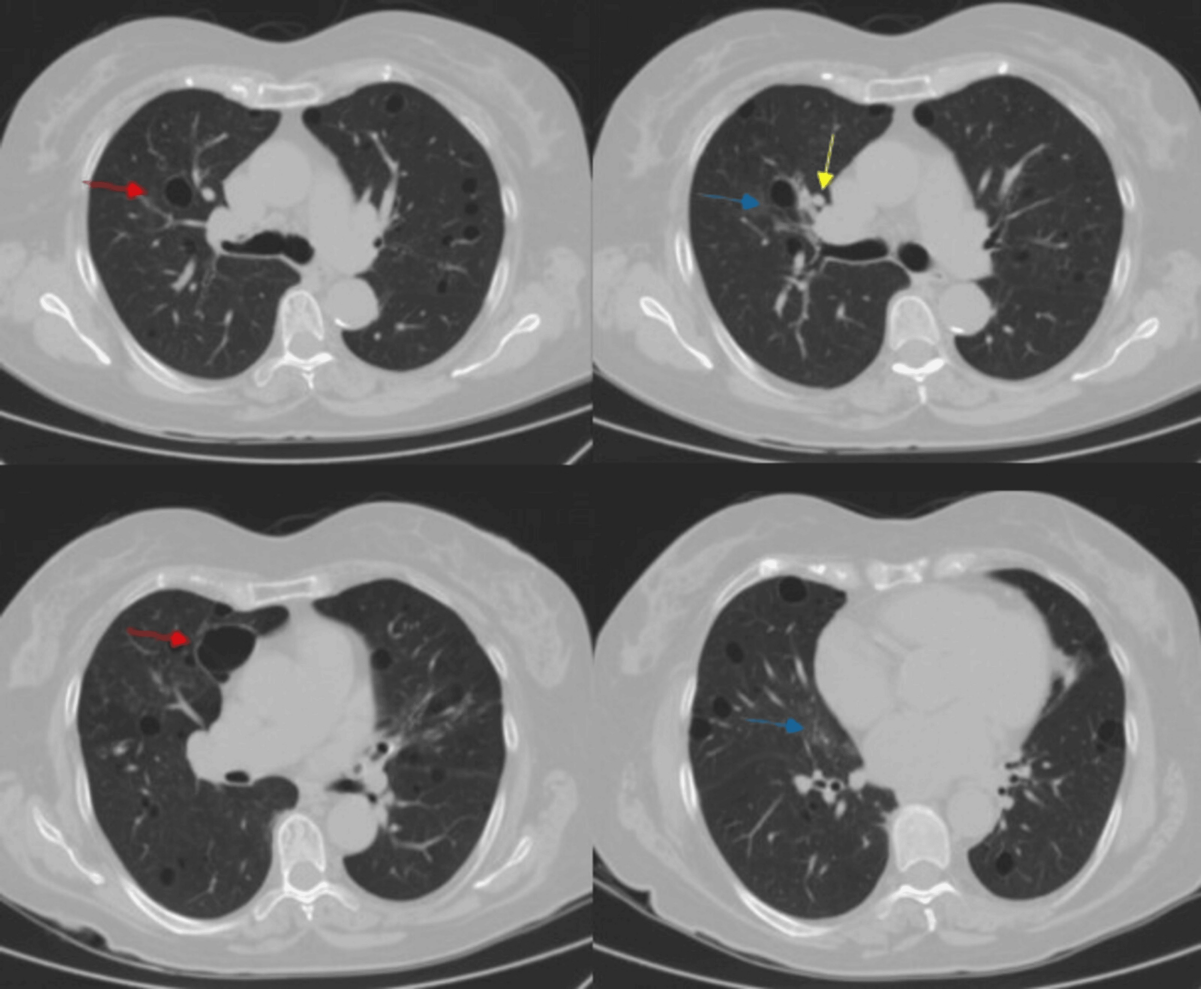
Chest CT scan obtained initially in 2022: pulmonary axial window. Multiple thin-walled cysts (red arrow) with dispersed bilateral distribution (some of them peribronchovascular) and ground-glass areas (blue arrow) in lung bases and middle lobe can be seen. Slight thickening of the bronchovascular interstitium (yellow arrow) can also be seen. There is no pleural or pericardial effusion, mediastinal adenopathy, or fibrosis.
